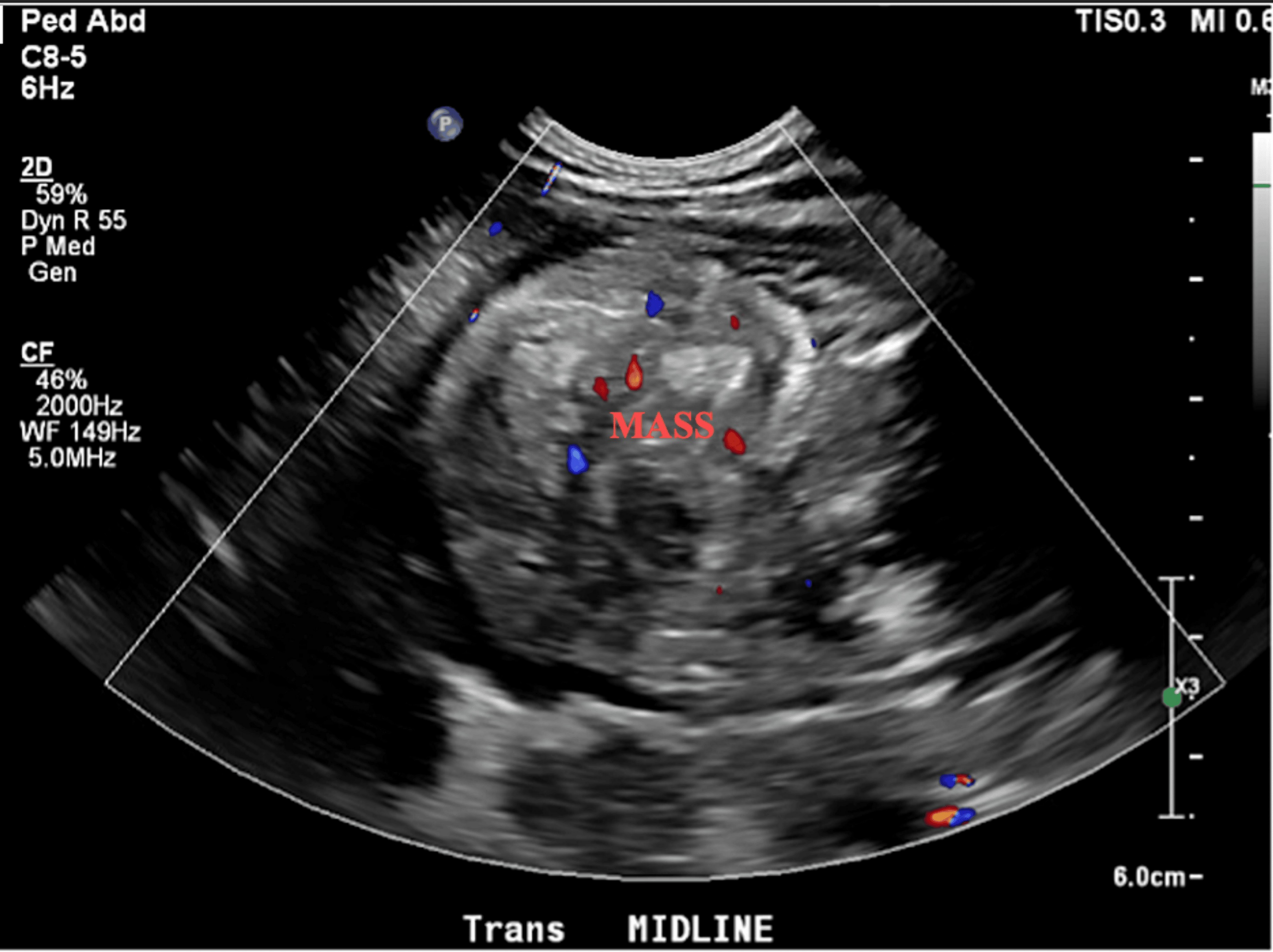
Abdominal ultrasound showing a gastric mass
